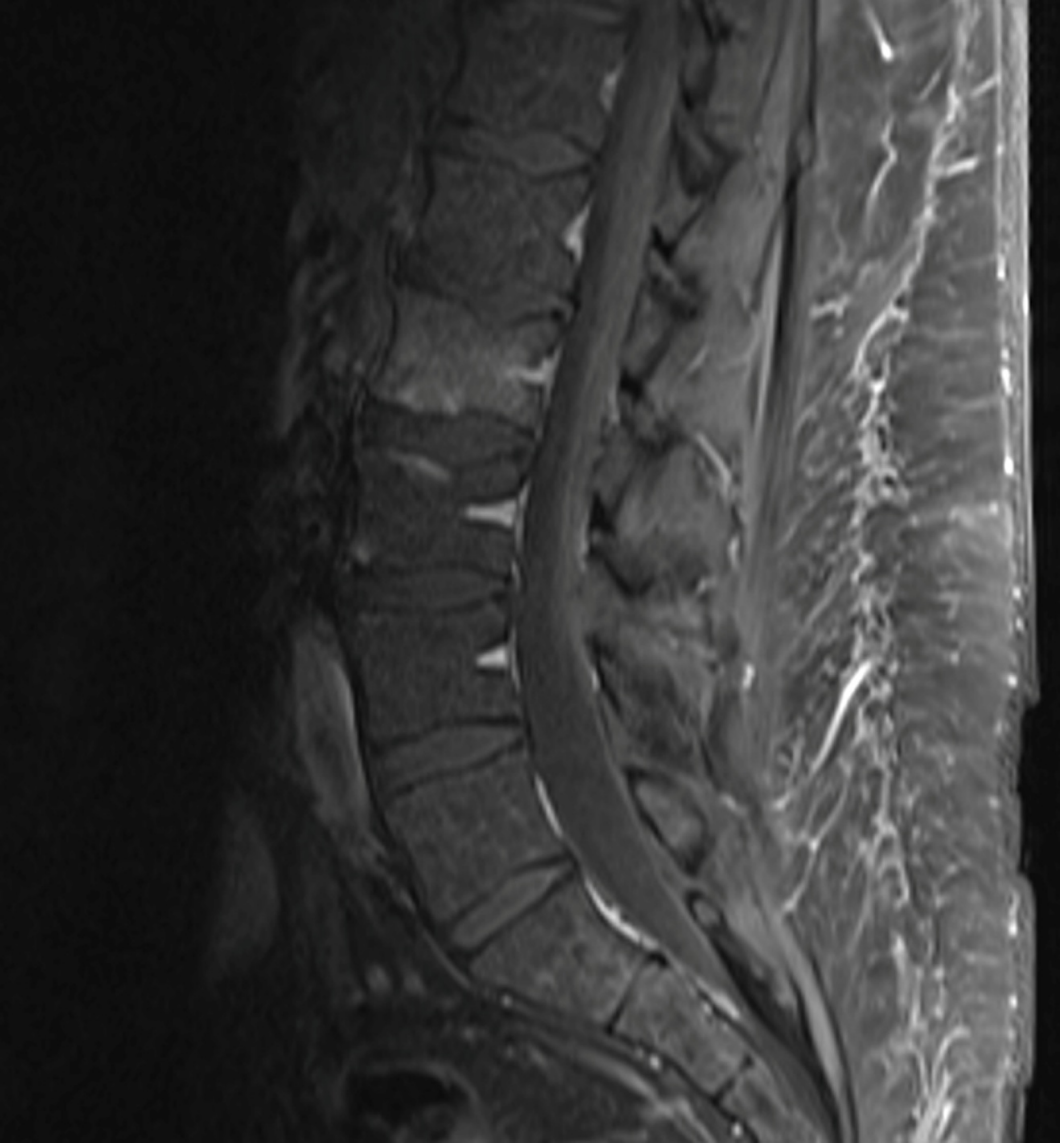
Initial MRI without contrast demonstrating foci of enhancement within the inferior L2 vertebral body.
